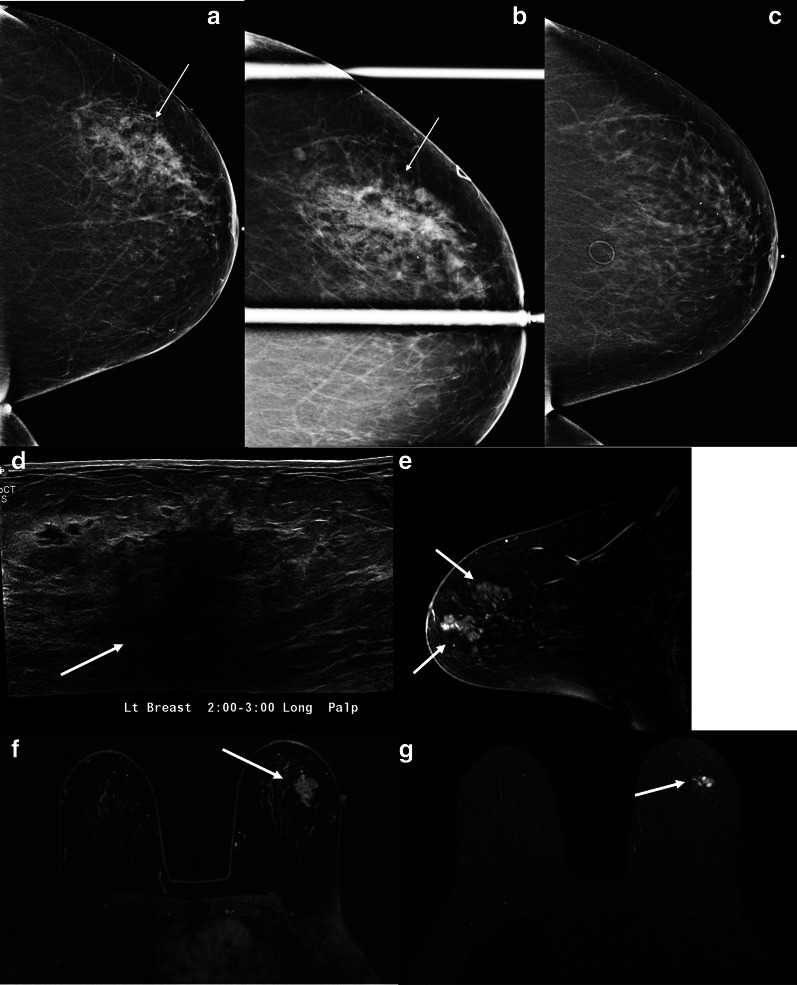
Suspicious presentation of pseudoangiomatous stromal hyperplasia. A 56-year-old woman with a new focal asymmetry in the outer breast that was identified on her (a) screening mammogram and persisted on (b) additional diagnostic spot compression views (arrows). The area was palpable by the patient and the clinician. The focal asymmetry had developed since her prior available mammogram performed 4 years earlier (c). Breast ultrasound (d) shows a corresponding irregular hypoechoic mass with associated posterior acoustic shadowing (arrow). Breast MRI sagittal post-contrast T1-weighted delayed images (e) and axial post-contrast T1-weighted images (f) show irregular enhancing masses (arrows). Corresponding T2 hyperintense cystic components with slit-like spaces (arrow) are identified (g), which favors PASH when present. Ultrasound-guided and MRI-guided biopsies were performed in this case and showed PASH. The area remained mammographically stable for over 4 years
